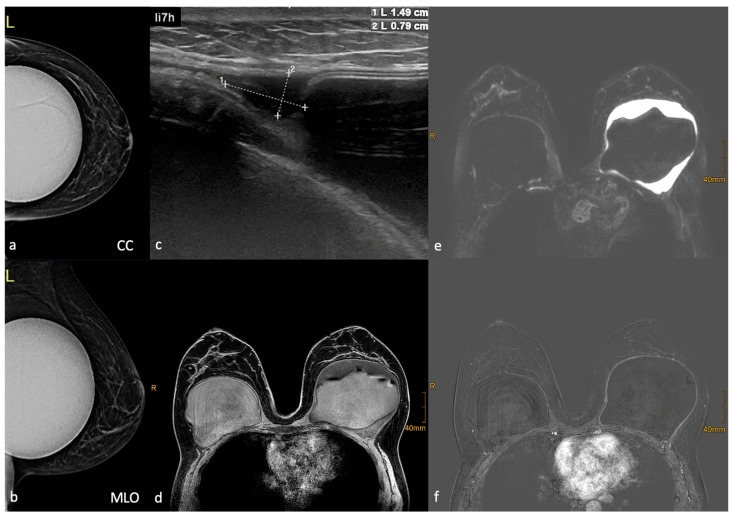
Imaging studies of the breast. (a,b) Two view 3D mammography (tomosynthesis) of the left breast shows isolated benign calcifications and an implant in situ. (c) Sonography of the left breast shows a homogeneous hypoechoic fluid collection around the subpectoral implant. (d) Axial T1 weighted MRI without contrast material showing bilateral implants. The left side shows fluid accumulation within the implant capsule and wrinkling of the implant. (e) Axial T2 fat-saturated MRI shows a high-signal fluid accumulation around the left implant, while the right implant remains unremarkable. (f) Axial T1 weighted MRI image with contrast material indicates capsule enhancement.
